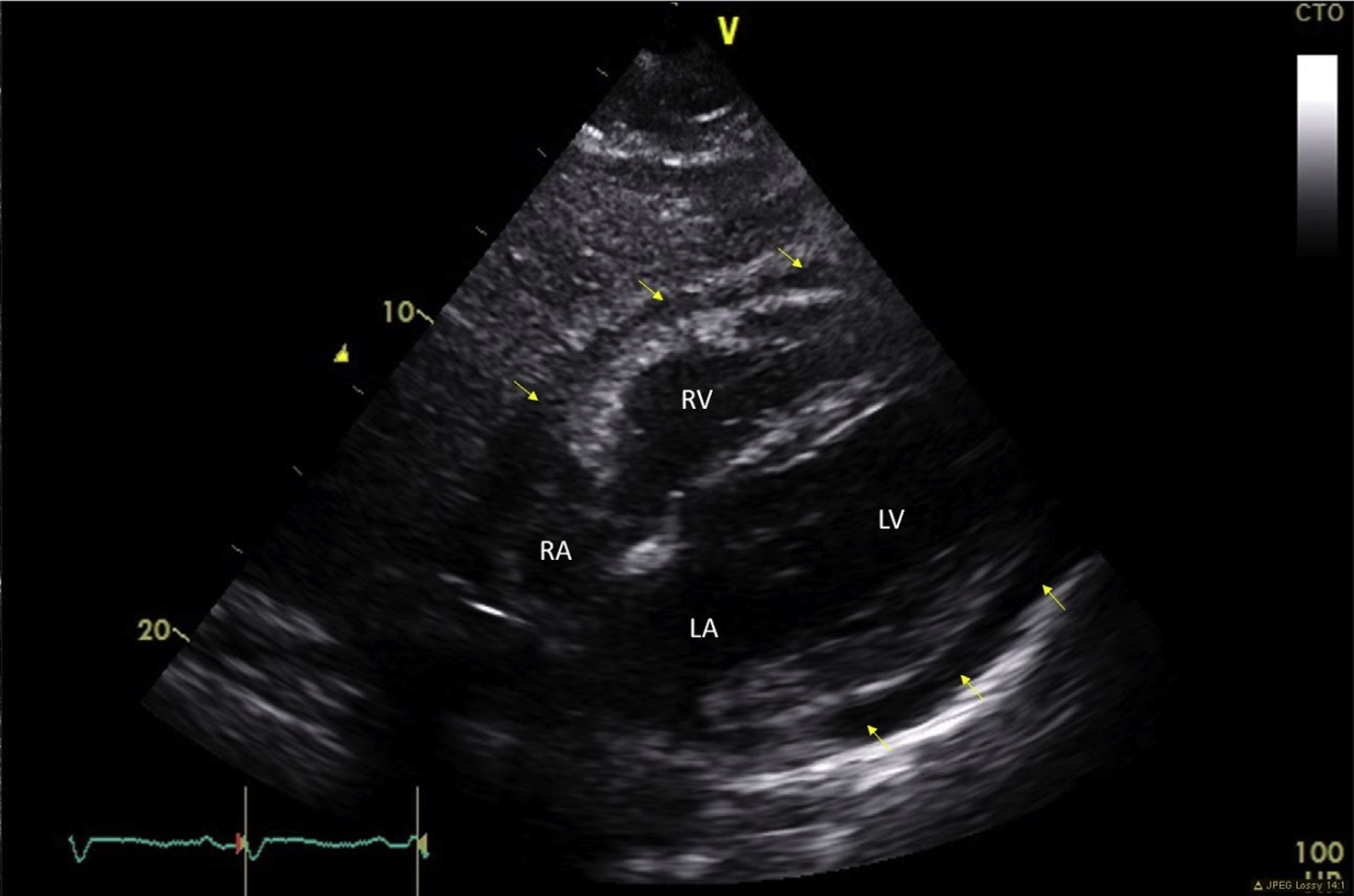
Transthoracic echocardiogram two-dimensional subcostal view of the heart showing moderate size circumferential pericardial effusion without tamponade (*yellow arrows*). *LA*, Left atrium; *LV*, left ventricle; *RA*, right atrium; *RV*, right ventricle.
